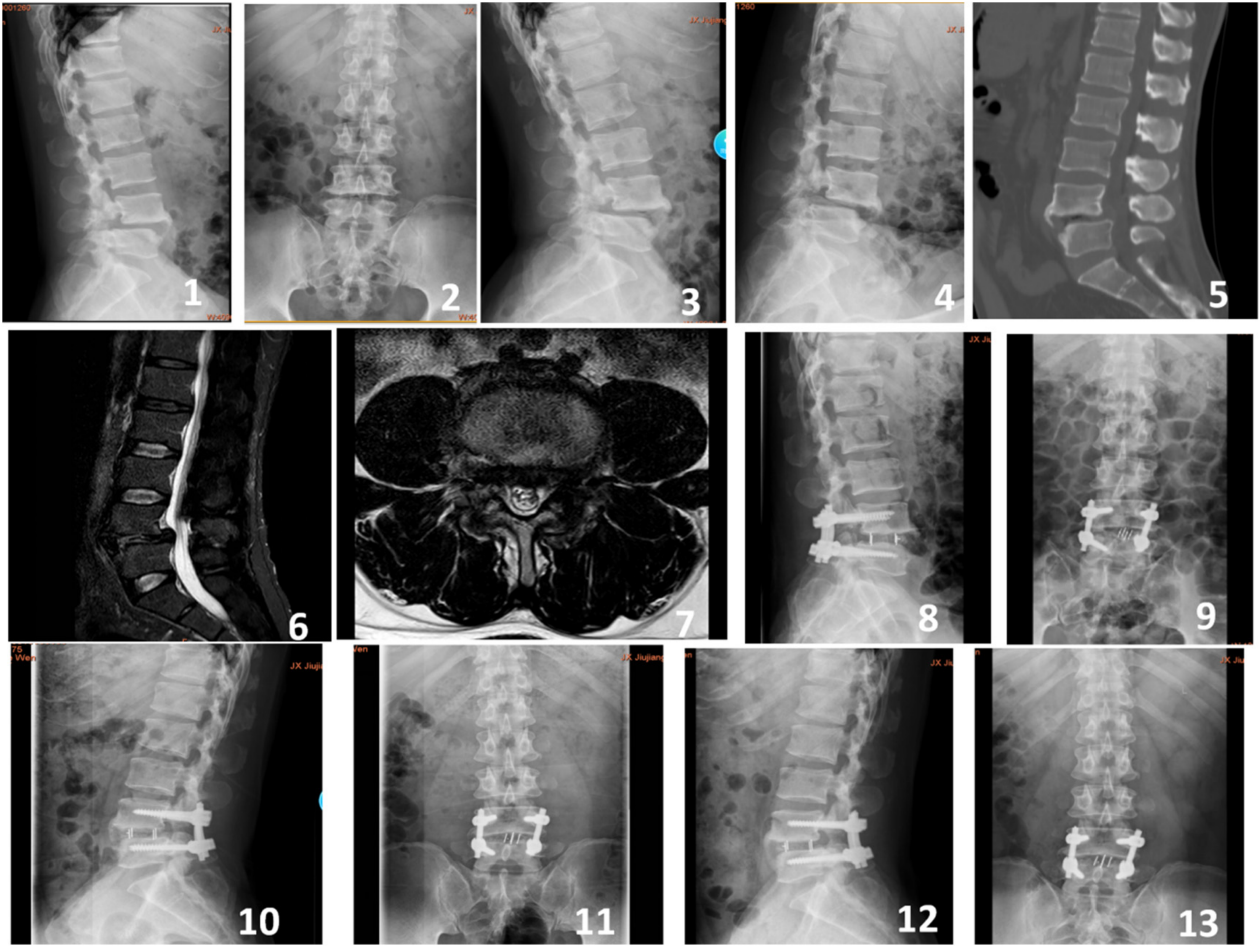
A 55-year-old male presented with recurrent lower back pain accompanied by intermittent claudication for 4 years. Preoperative imaging included lateral, anteroposterior, and dynamic flexion-extension lumbar radiographs (Figures 1-4), preoperative CT scan (Figure 5), and preoperative MRI (Figures 6-7). Postoperative imaging at 3 days following the open surgery included lateral and anteroposterior lumbar radiographs (Figures 8-9). Follow-up imaging at 3 months (Figures 10-11) and 12 months (Figures 12-13) postoperatively showed good bony fusion on lateral and anteroposterior lumbar radiographs.
